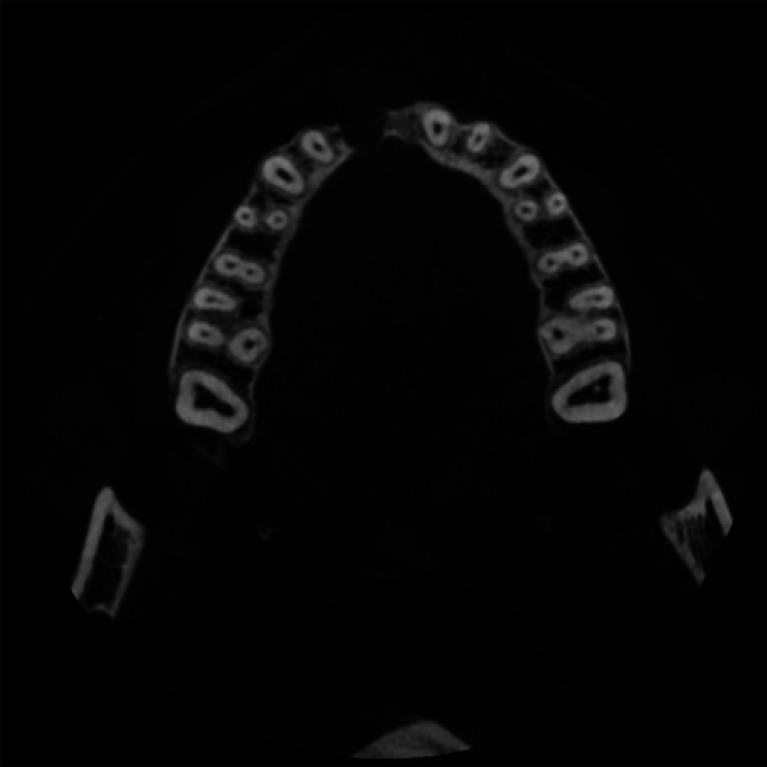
CBCT (Cone-Beam Computed Tomography) in the axial plane revealed two-rooted maxillary first premolars (arrows indicate the examined teeth)
